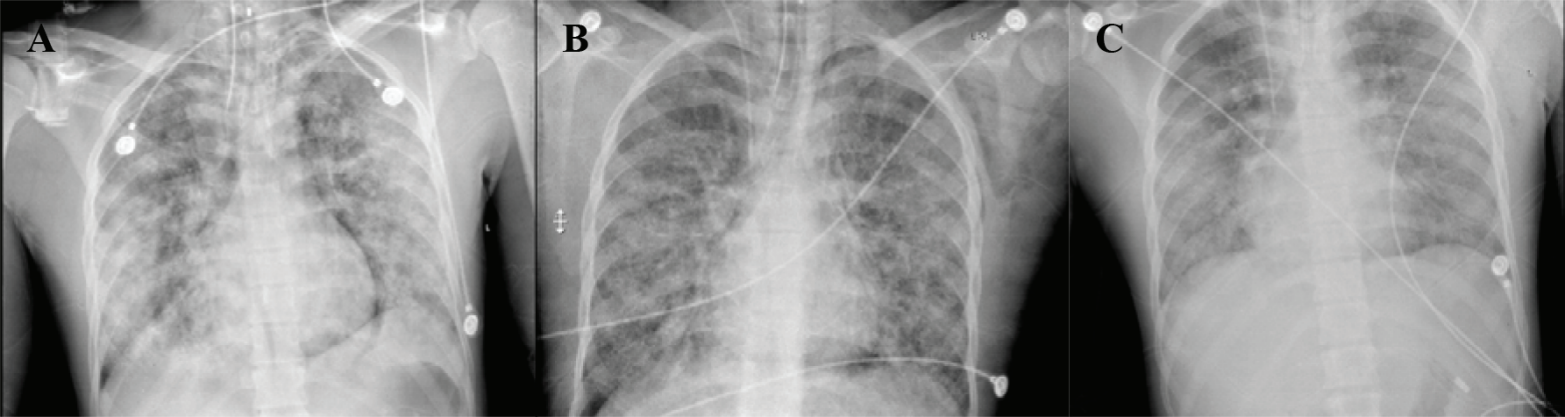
Serial images of daily chest radiographs taken during patient’s stay at the ICU. A) image on day 1st;B) image on day 2ndafter 16 hours of prone positioning; C) image on day 3
